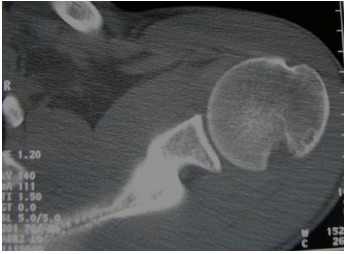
A transverse plane CT image demonstrating a large Hill-Sachs lesion of the humeral head.
